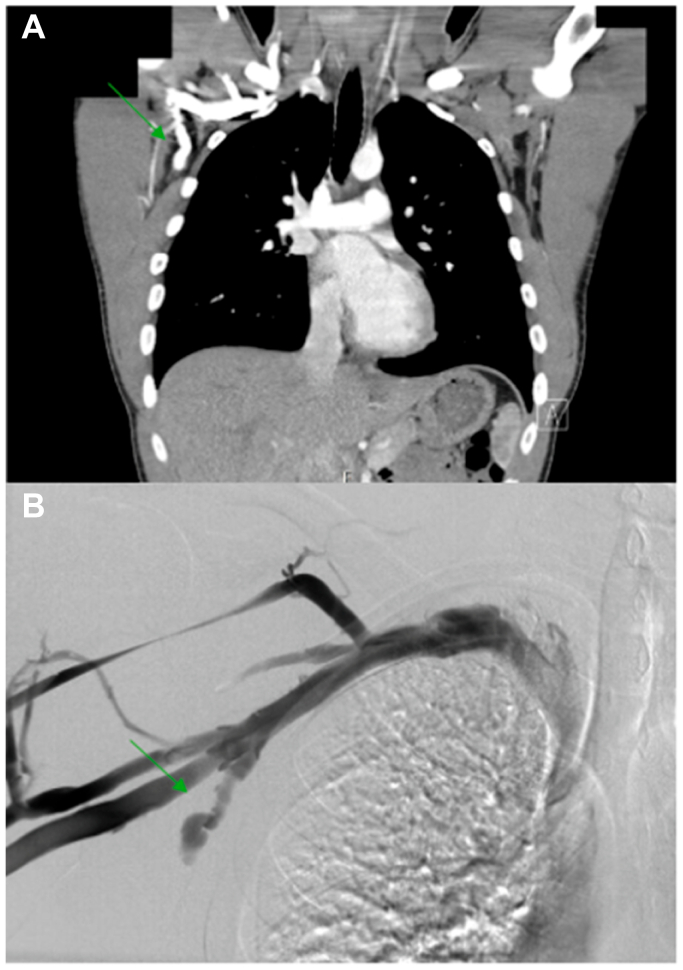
Preoperative (A) computed tomography (CT) angiogram, coronal view, and (B) venogram demonstrating the chest wall varix (*green arrows*).
